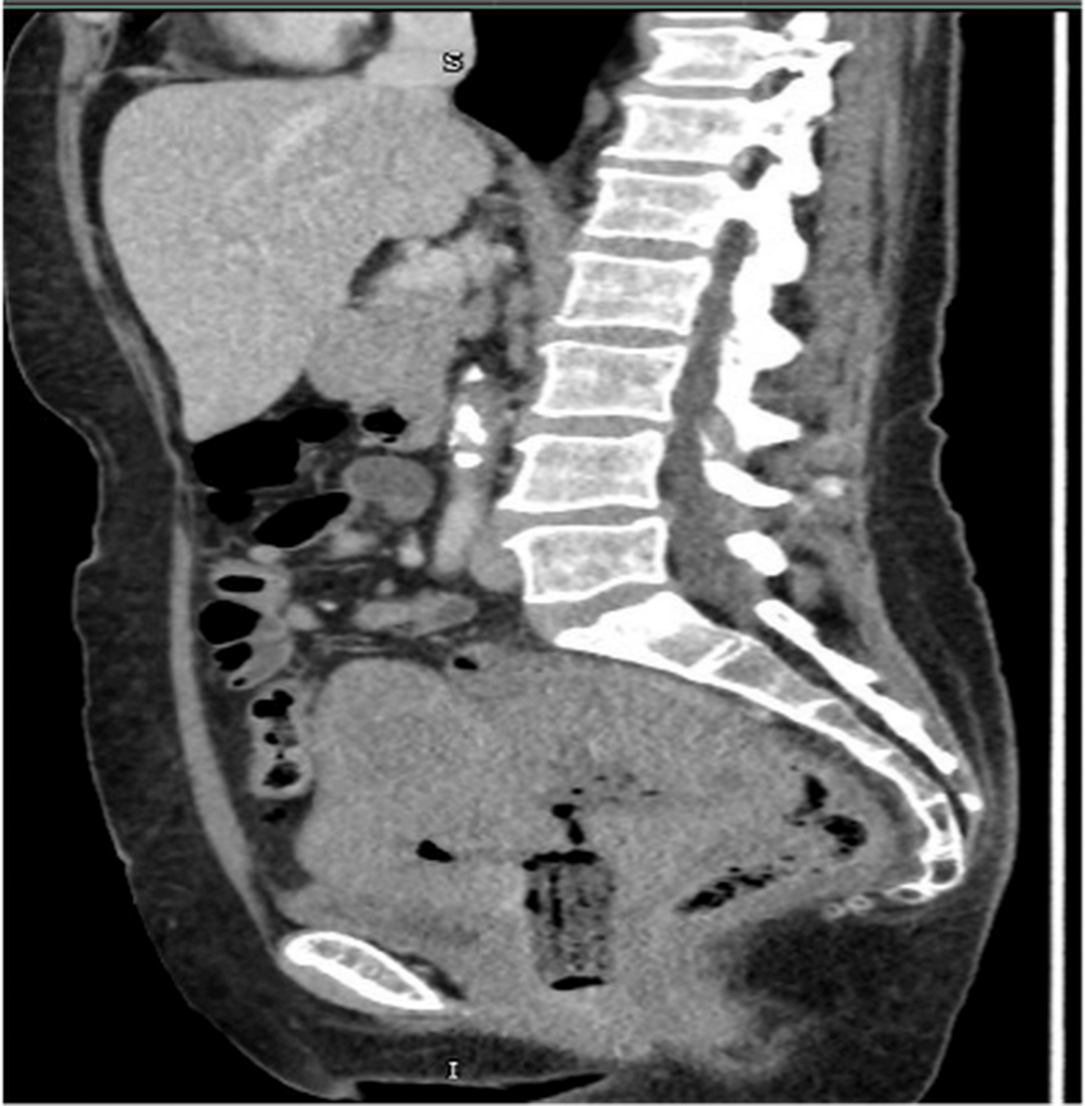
CT (sagittal view) showing a huge pelvic mass that is inseparable from the bladder and rectosigmoid.
